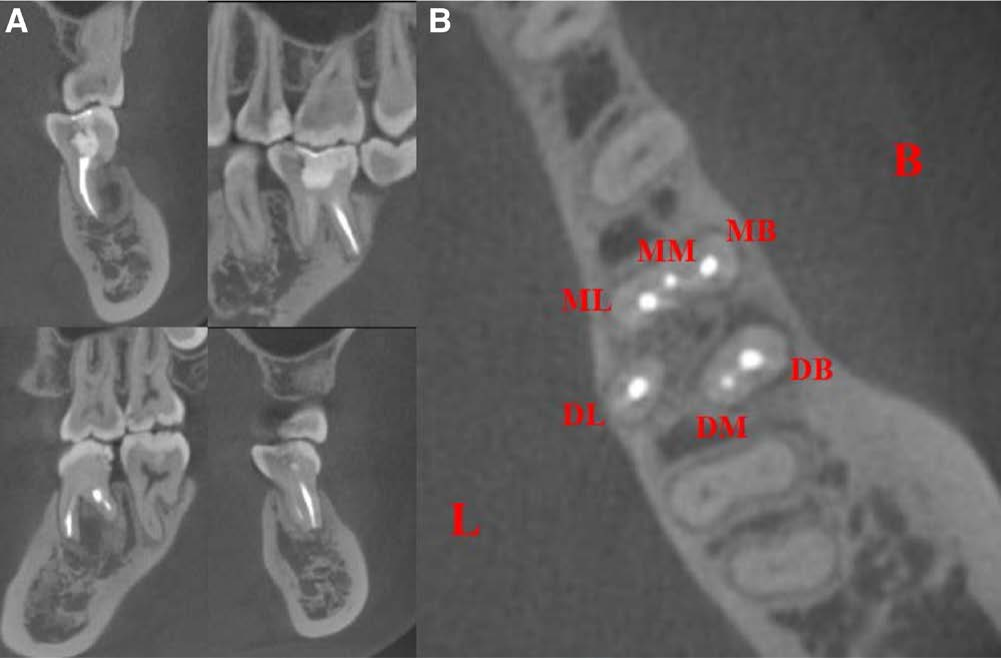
CBCT images of tooth 36 at the 3-month follow-up visit. (A) A significantly decreased apical lesion. (B) Cross-sectional view. CBCT = cone-beam computed tomography.
